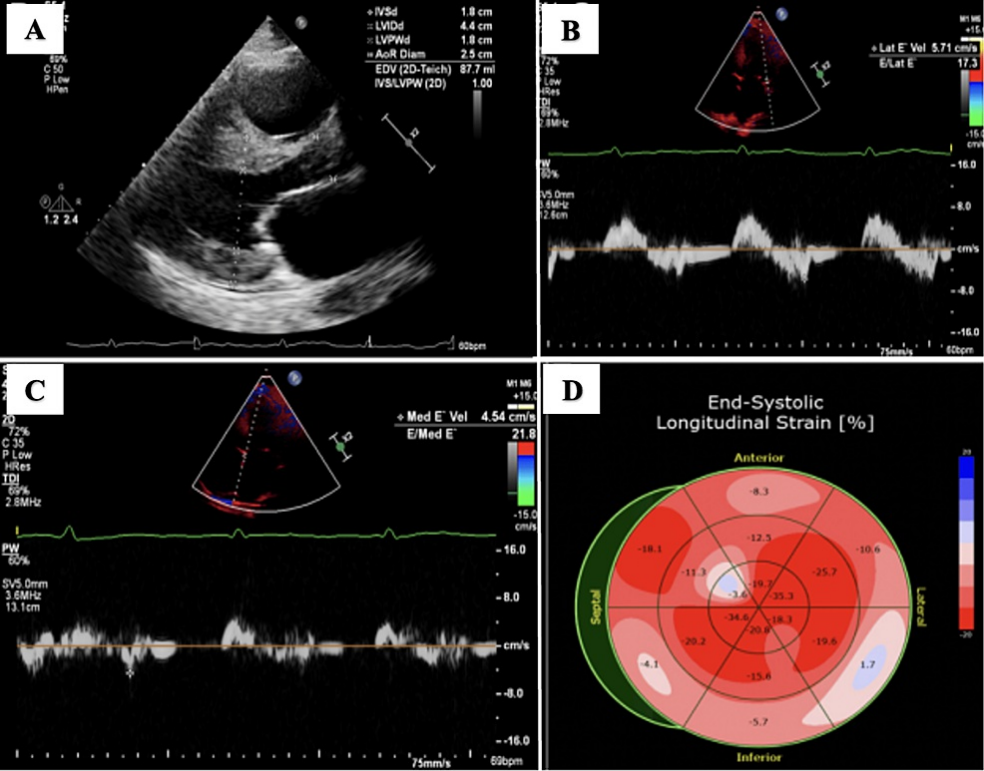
Transthoracic echocardiography (TTE) images A: Parasternal long-axis view showing severe concentric left ventricular hypertrophy. B: Tissue Doppler showing reduced lateral E’ velocity of 5.71 cm/s and elevated E/lateral E’ of 17.3. C: Tissue Doppler showing reduced medial E’ velocity of 4.54 cm/s and elevated E/medial E’ of 21.8. D: Global longitudinal strain score of -16% with apical sparing.
